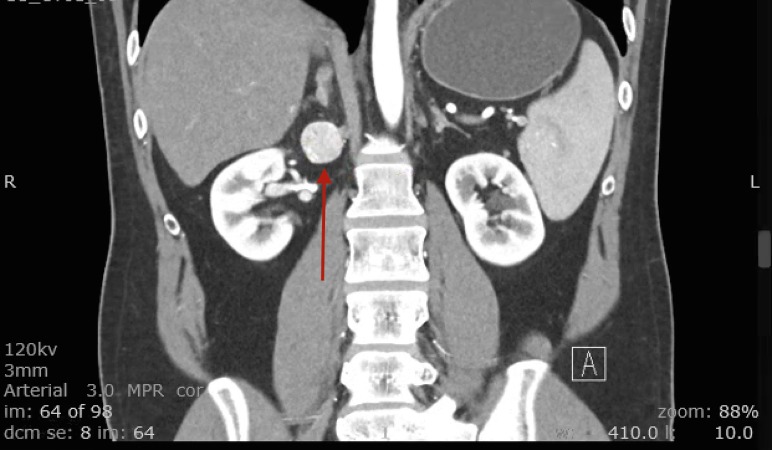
CT Scan Patient One Red arrow shows hyper-intense lesion medial to the right kidney, suggestive of a paraganglioma
